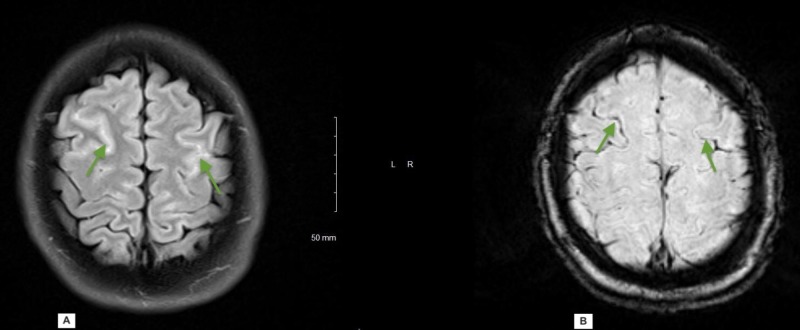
Axial sections of MRI brain T2 FLAIR (A) demonstrating hyper intensity in the bilateral frontal region and prominent blood vessels on the corresponding SWI sequence (B) MRI: magnetic resonance imaging; FLAIR: fluid-attenuated inversion recovery; SWI: susceptibility-weighted imaging
